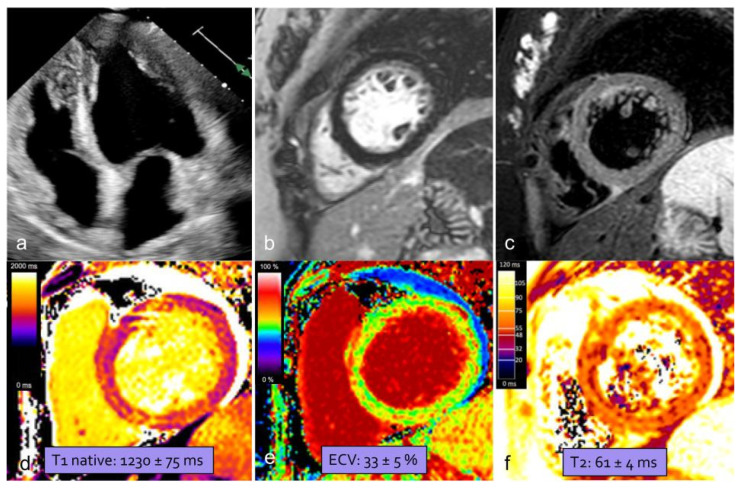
A 38-year-old woman presented with chest oppression and hypertension eight days after delivery. TTE (a) showed decreased LVEF (45%) and global left ventricle hypokinesia. CMR confirmed left ventricle disfunction, but no focal delayed enhancement was identified on LGE imaging (b) and STIR images (c) showed faint and diffuse hyperintensity. Quantitative mapping showed high global myocardial native T1, ECV, and T2 values ((d–f): mean value of the basal segments shown in the Figures). TTE: transthoracic echocardiogram; LVEF: left ventricular ejection fraction; CMR: cardiac magnetic resonance; LGE: late gadolinium enhancement; STIR: short tau inversion recovery; and ECV: extracellular volume.
